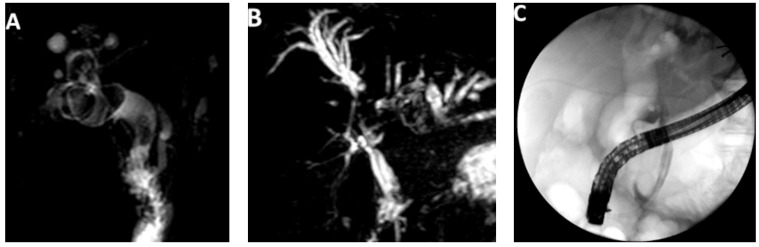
Cases of difficult biliary stones not amenable to conventional ERCP treatment. (A,B) Magnetic Resonance Images showing complex bile duct stones with large signal defects extending to the hilum and intrahepatics; (C) cholangiogram showing a very large common bile duct stone. All cases were successfully managed with single-operator cholangioscopy-assisted electrohydraulic lithotripsy.
